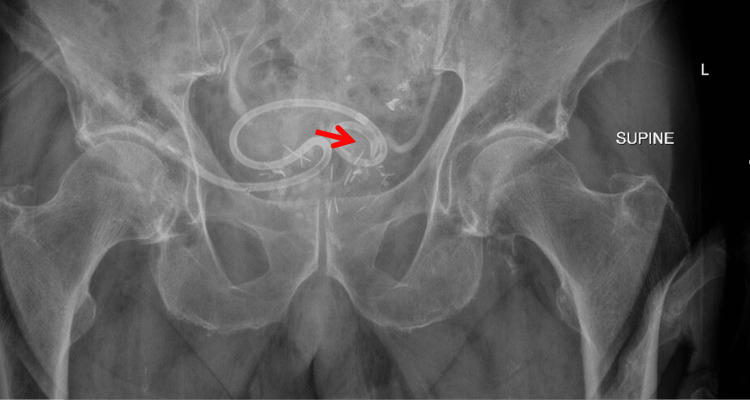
Pelvic X-ray following suprapubic catheter placement Pelvic radiograph demonstrating appropriate positioning of the pigtail catheter within the bladder, with visualization of the characteristic coiled tip (arrow)
